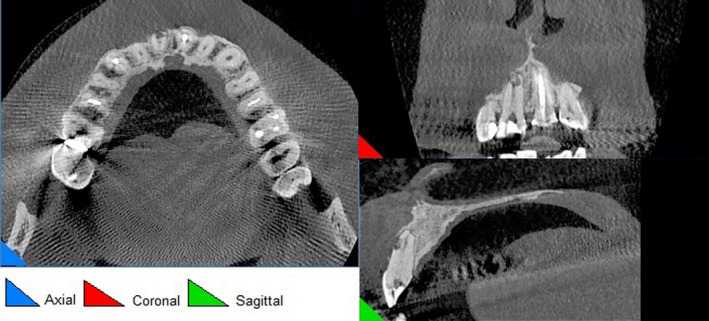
Coronal, sagittal, and axial view on CBCT
